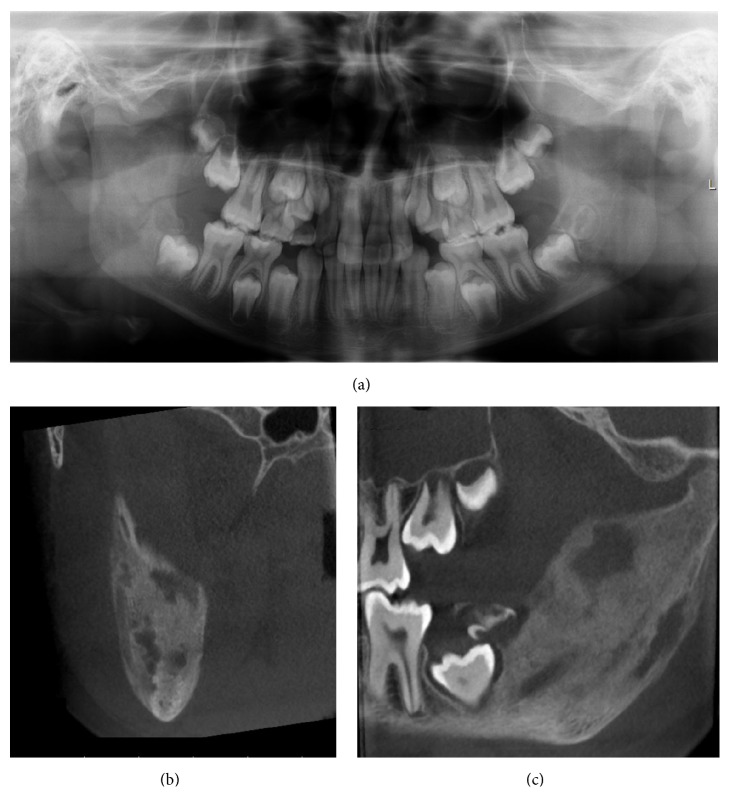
Preoperative radiographs and CBCT of patient case 2 with PCO. (a) Panoramic radiograph; (b and c) CBCT. Coronal and sagittal sections of sclerotic mandible with new bone formation and lytic lesions at the right posterior site.
